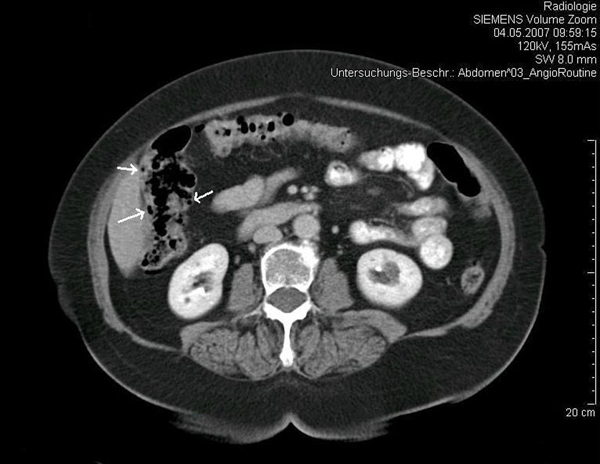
Abdominal computed tomography scan. Arrows show intramural air in the ascending colon.
